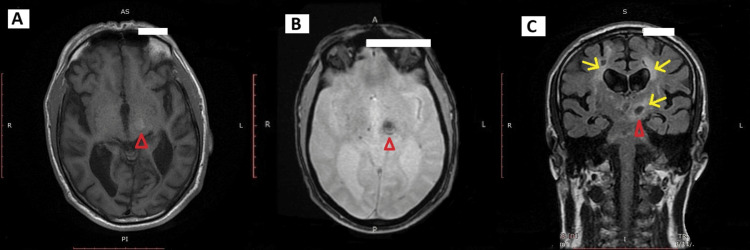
MRI of the brain demonstrating thalamic hemorrhage and chronic small vessel ischemic changes Multiplanar brain MRI demonstrating a 1.3 cm subacute hemorrhagic lesion centered in the left thalamus and superior cerebral crus (red arrowhead), appearing:
